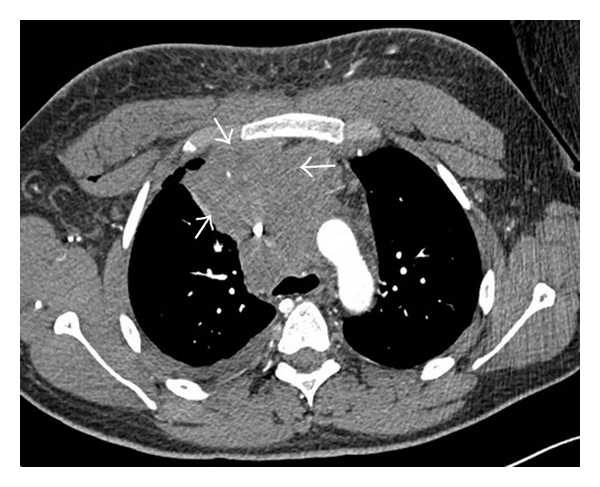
Pretreatment computed tomography of the thorax demonstrating a large anterior mediastinal mass (see arrows).
